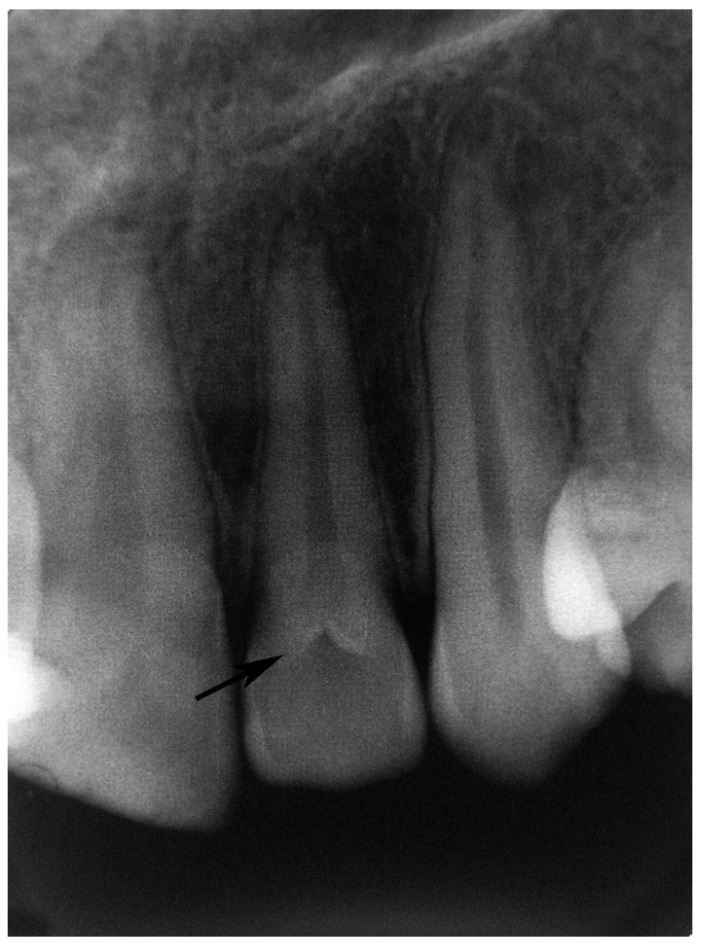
Periapical radiography shows a type I dens invaginatus according to the classification of Oehlers (arrow shows dens invaginatus).
